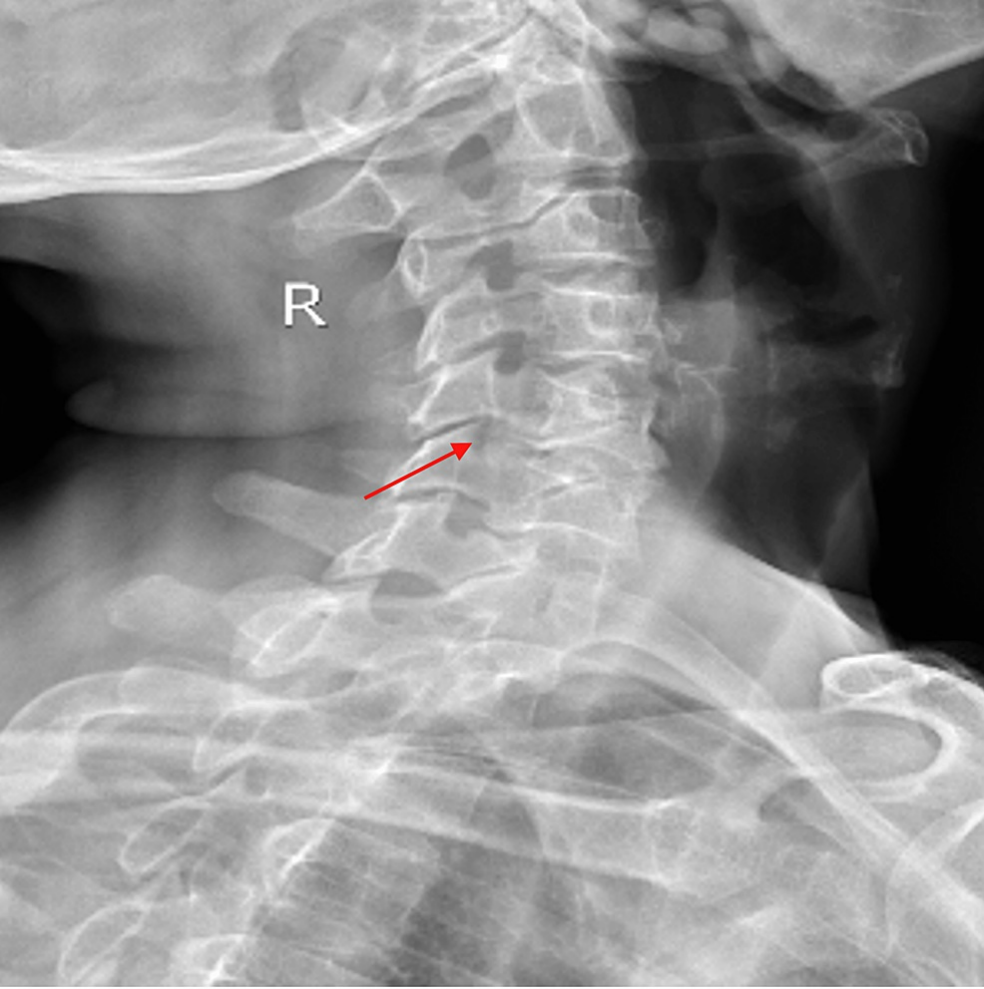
Sample X-ray image of cervical spinal stenosis
